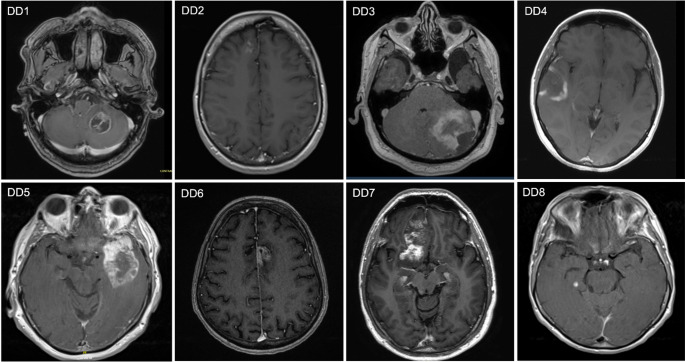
Contrast-enhanced T1-weighted MRI of *IDH*-WT, *H3*-WT, *TERT*p-WT astrocytic tumors without genomic GBM-defining alterations (*n* = 8)
